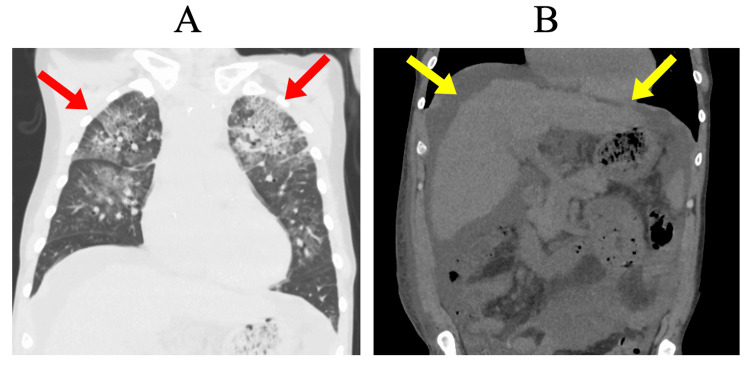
Non-contrast chest and abdominal CT on the initial examination (A) Non-contrast chest CT showing pulmonary infiltrates (red arrows). (B) Non-contrast abdominal CT showing liver cirrhosis characterized by irregular and nodular liver margins (yellow arrows) and ascites. CT, computed tomography.
